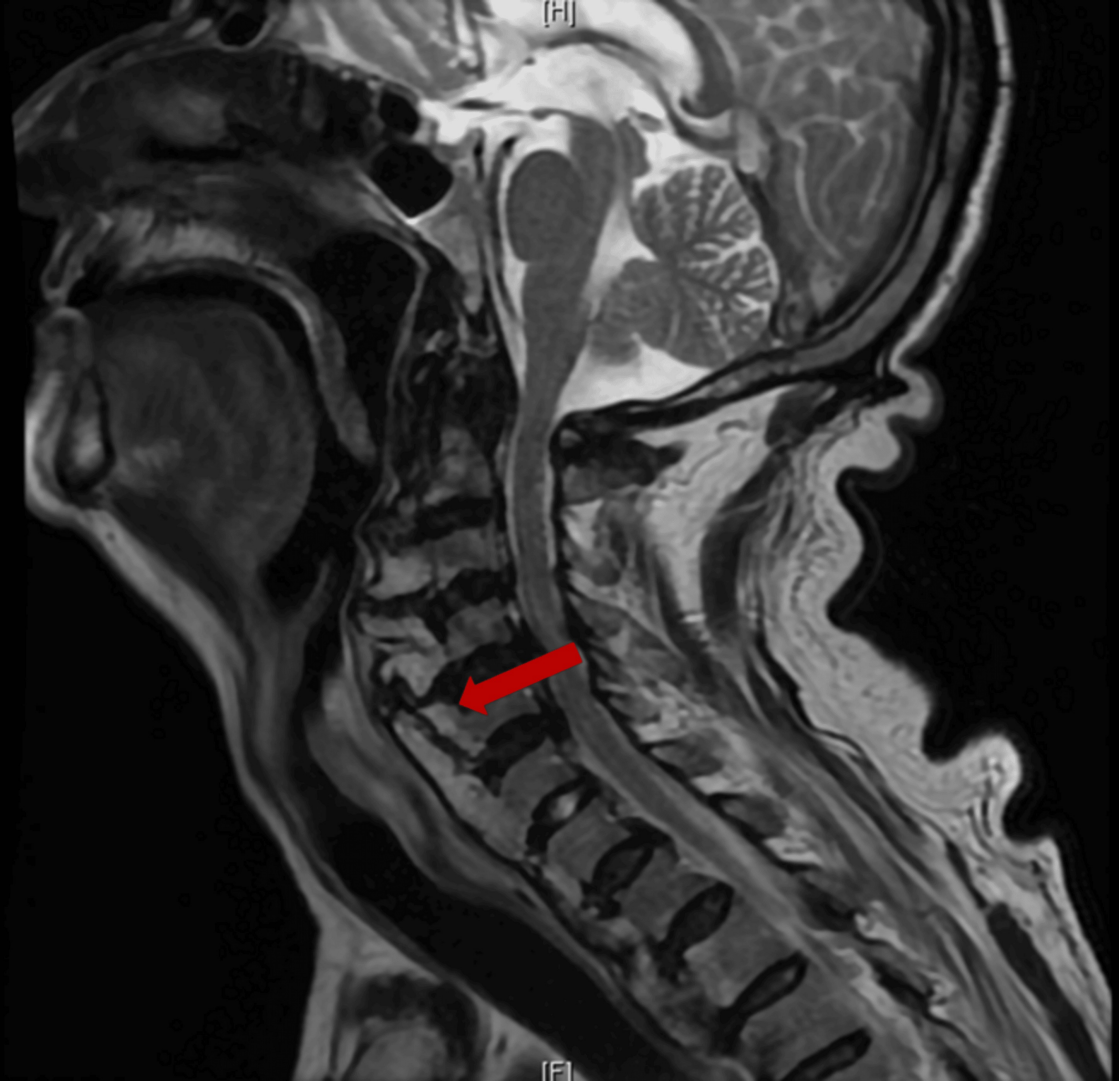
MR imaging showing exuberant anterior osteophyte causing significant upper airway narrowing and esophagus compression (red arrow)
